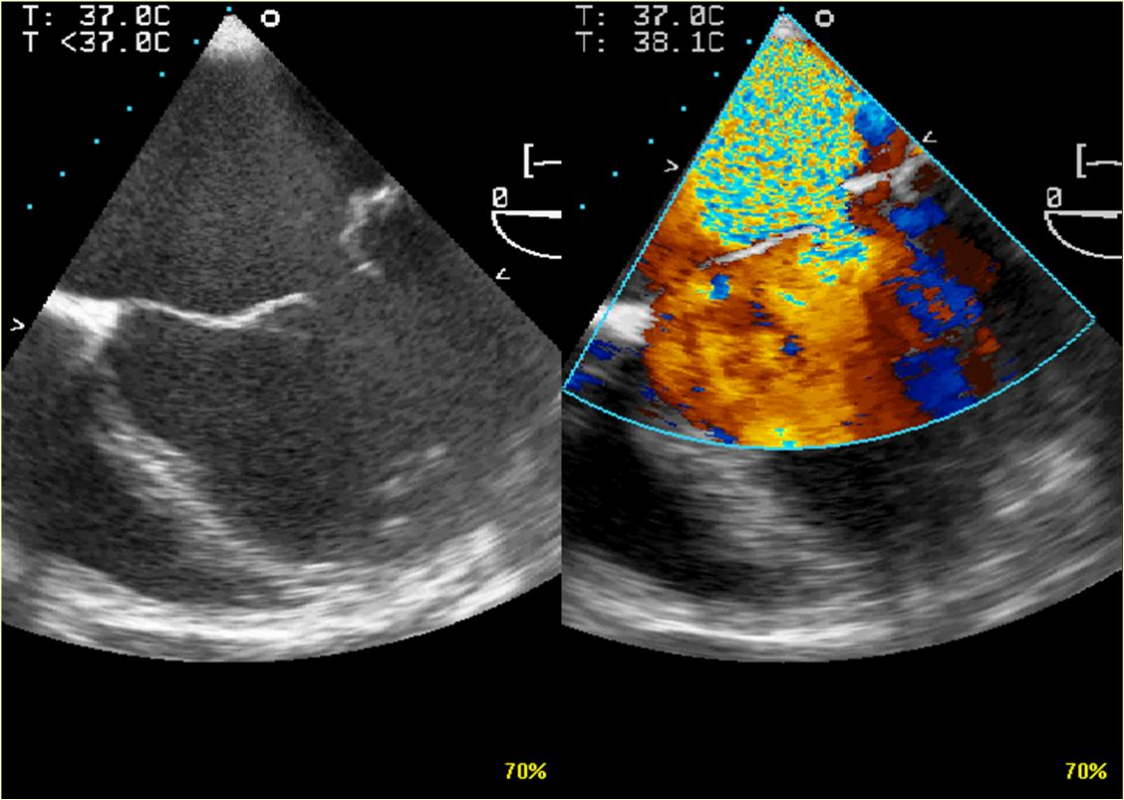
Transesophageal echocardiogram: 2D (A) and color
